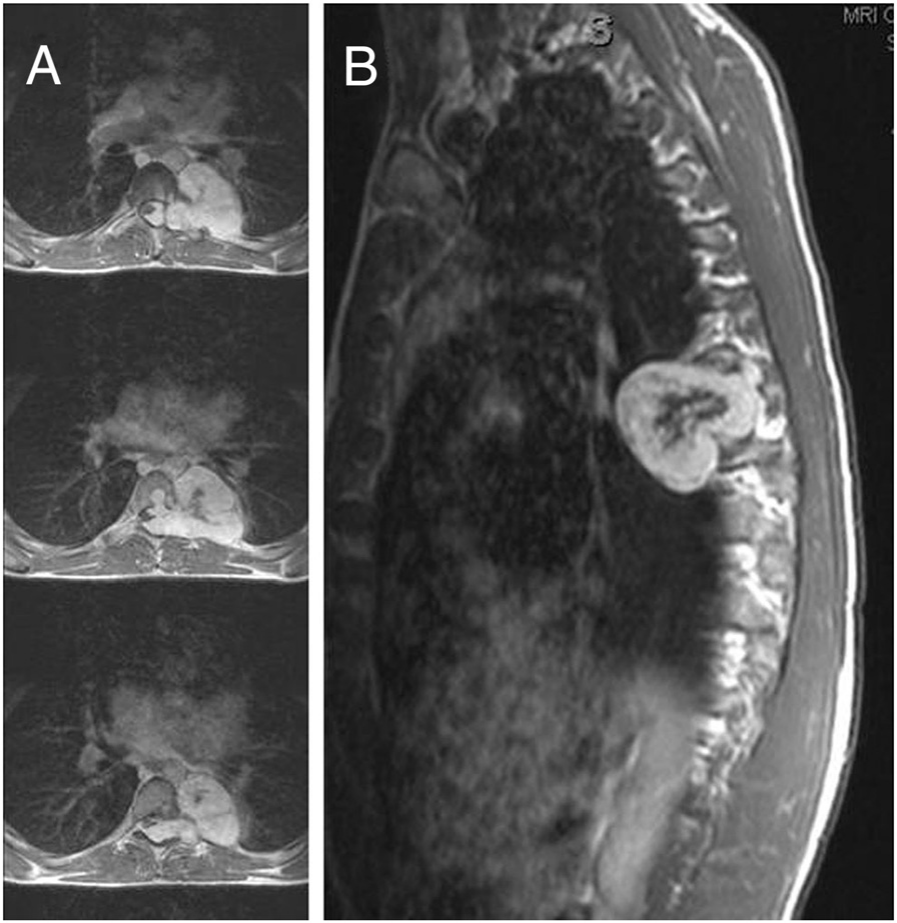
Preoperative thoracic magnetic resonance imaging (MRI) scan demonstrates a giant invasive spinal schwannoma with spinal cord compression at T7-8 level. (A) axial- and (B) sagittal-enhanced T1-weighted MRI scan also shows extension through the left T7-8 neural foramen into the retropleural space.
